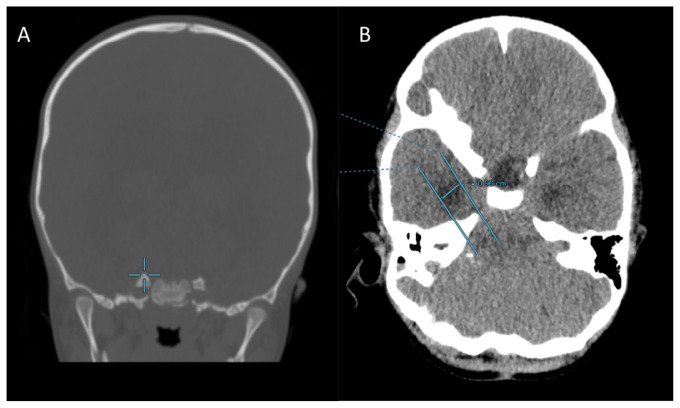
CT images of the similar 3 years old child with grade 4 medulloblastoma as in the case in Figure 4
